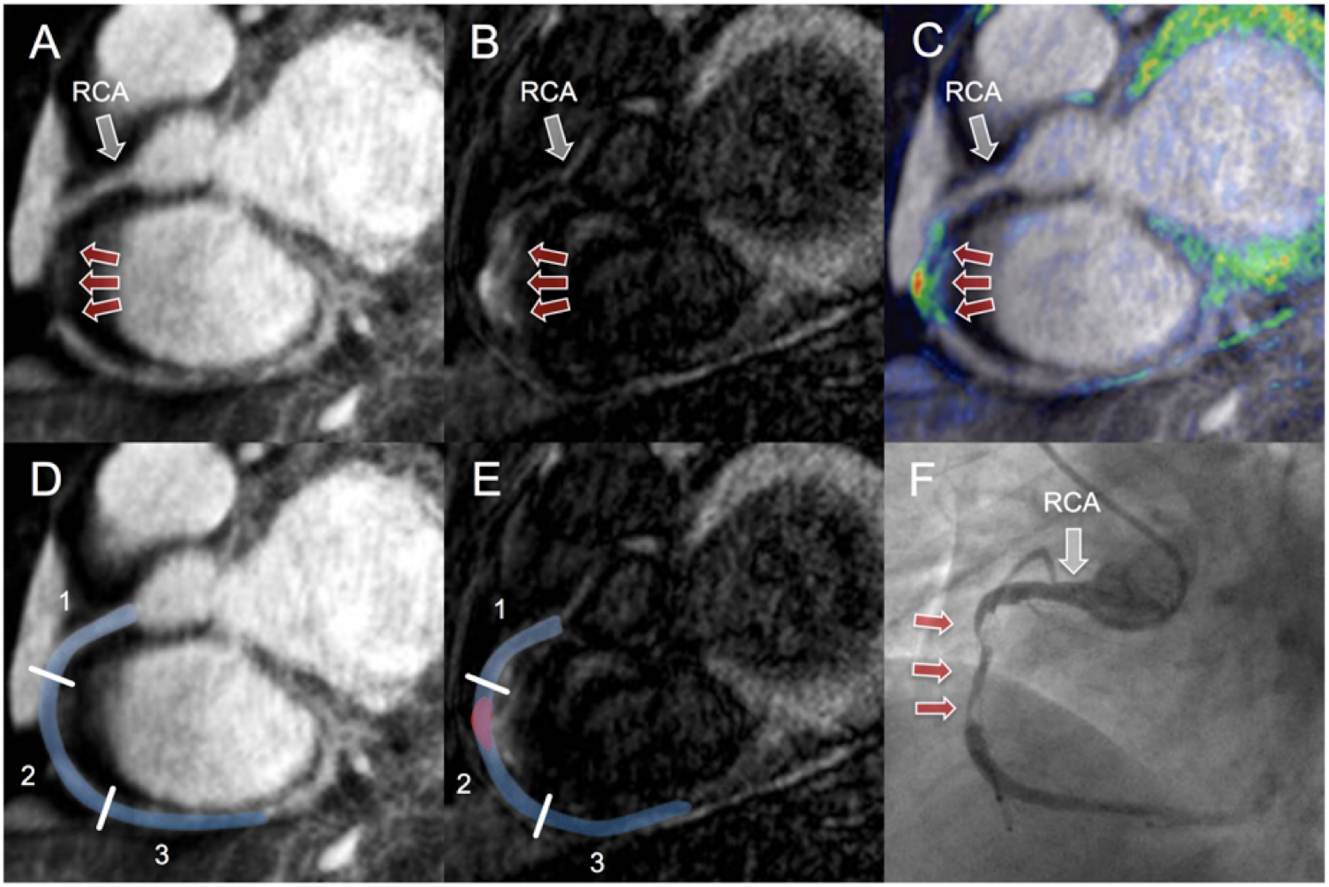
CE-MRCVI findings in a patient with troponin positive ACS in the RCA. Coronary MRA (A) of a 48-year-old male with troponin positive ACS showed decreased vessel lumen size of the mid RCA (red arrows). To highlight the relationship between CE-MRCVI (B) and morphology (A), images were fused in a way similar to PET/CT (C). CE-MRCVI displays high signal intensity (red arrows) within the mid RCA. Analysis of signal enhancement on CE-MRCVI (E, red) suggestive for culprit lesion yielded a contrast-to-noise ratio (CNR) of 12.7. Corresponding XCA (F) confirmed CE-MRCVI findings with mid RCA de-novo lesion (pre-treatment stenosis of 75–94%). MRA: magnetic resonance angiography, CE-MRCVI: contrast enhanced magnetic resonance coronary vessel wall imaging, PET/CT: positron emission tomography/computed tomography. CE-MRCVI:contrast enhanced magnetic resoance coronary vessel wall imaging, ACS: acute coronary syndrome, RCA: right coronary artery, MRA: magnetic resonance angiography, XCA: x-ray coronary angiography, PET/CT: positron emission tomography/computed tomography.
